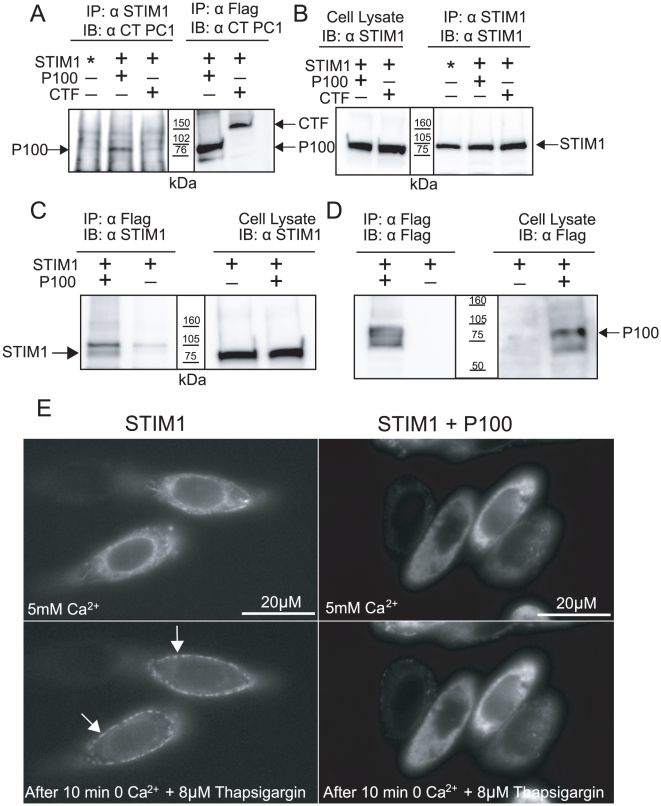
P100 physically interacts with STIM1. (A) CHO cells transiently transfected with STIM1 and either P100 or CTF construct; immunoprecipitated with anti-STIM1 antibody, then probed with anti-CT;or immunoprecipitated with flag conjugated beads then probed with the anti-CT antibody to verify transfection of PC1 products. * denotes endogenous STIM1 only. (B) STIM1 expression was verified by probing the same lysate and immunoprecipitate used in A and B with anti-STIM1 antibody. Blots representative of at least 3 experiments. (C) To confirm the pull down of P100 by STIM1, the reverse was attempted, pulling down STIM1 with P100. CHO cells were again transiently transfected with STIM1 and P100 or STIM1 alone. The Flag tagged P100 was immunoprecipitated with flag conjugated beads then probed with anti-STIM1 antibody. The expression of STIM1 was verified by probing the lysate directly with the anti-STIM1 antibody. (D) The expression of P100 was confirmed by probing the lysate and the immunoprecipitate with anti-Flag. Blots representative of at least 3 experiments. (E) CHO cells transfected with YFP-STIM1 and P100 (right) or the empty plasmid (left) and imaged first in a 5 mM Ca^2+^ bath solution (top) then again after a 10 min incubation in a zero Ca^2+^ bath with 8 µM thapsigargin (bottom). P100 retards the peripheral YFP puncta formation.
